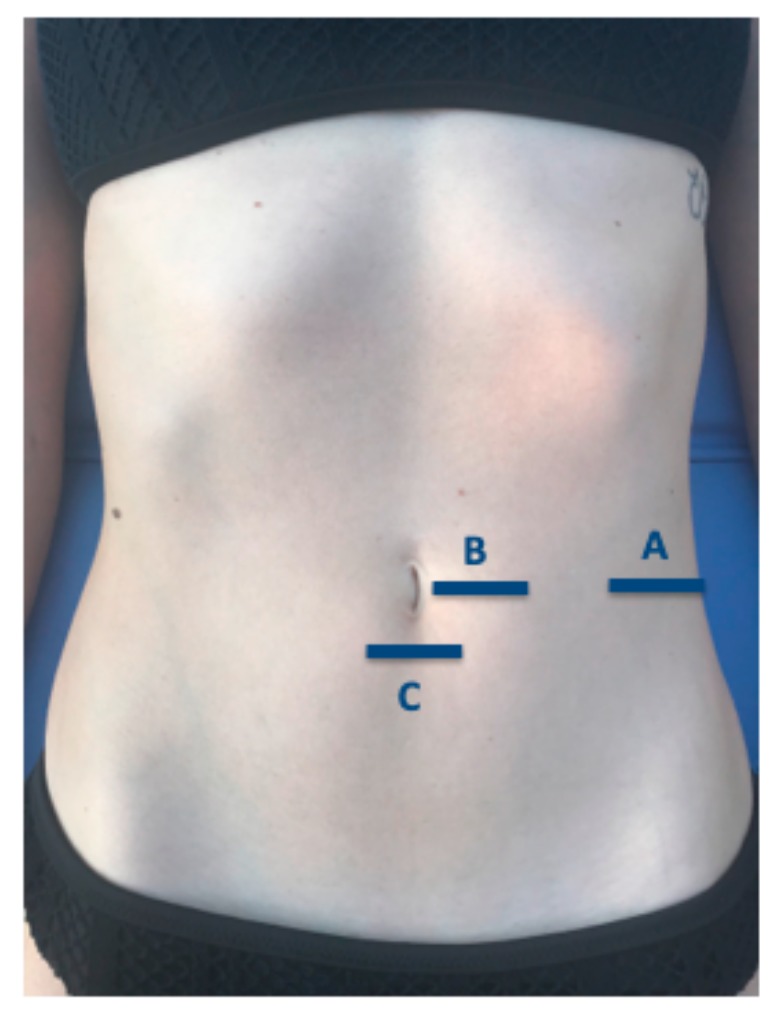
Probe locations during ultrasound evaluation of the abdominal wall muscles.
